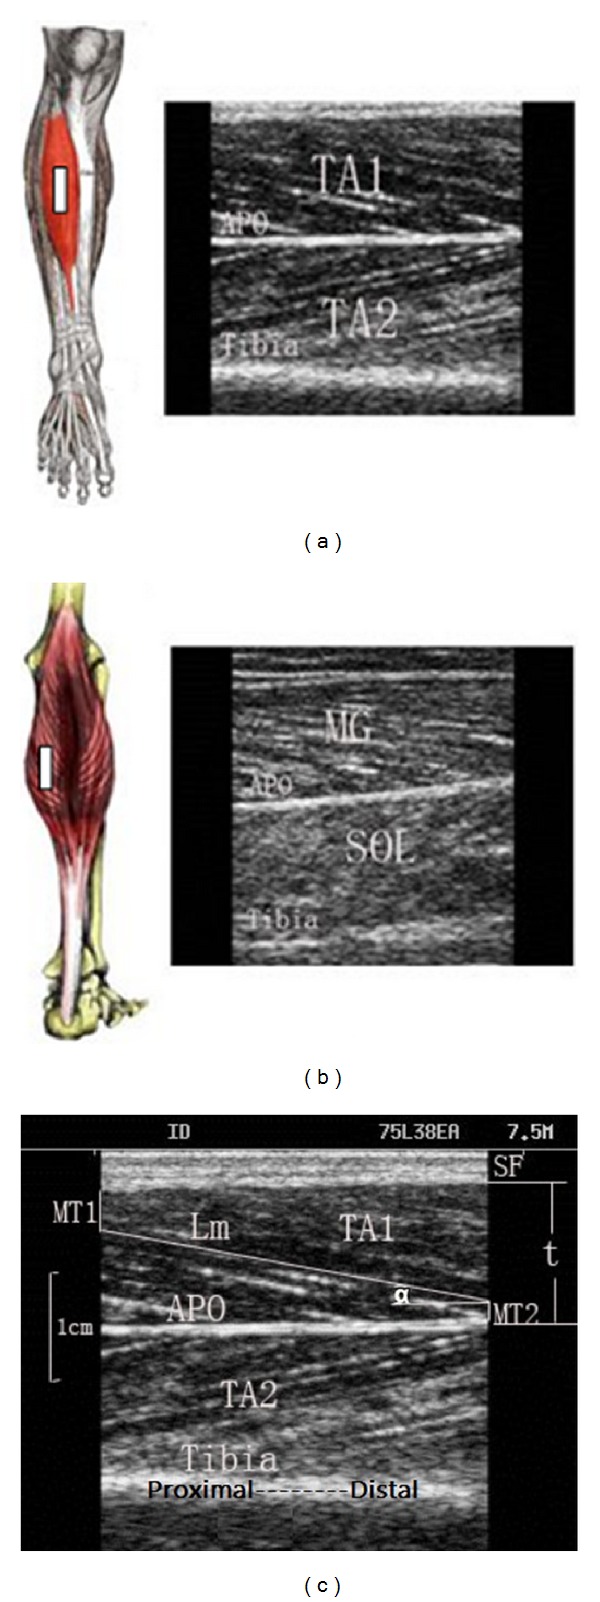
Probe positions on the measured muscles and typical ultrasound images for measurement on (a) TA and (b) MG. (c) Demonstration of the labels for muscle parameters. The bright fringe in the lower region of the image shows the muscle-tibia boundary. Aponeurosis (APO) is the boundary between the superficial and deep layer of TA. SF is subcutaneous fat. *L*
